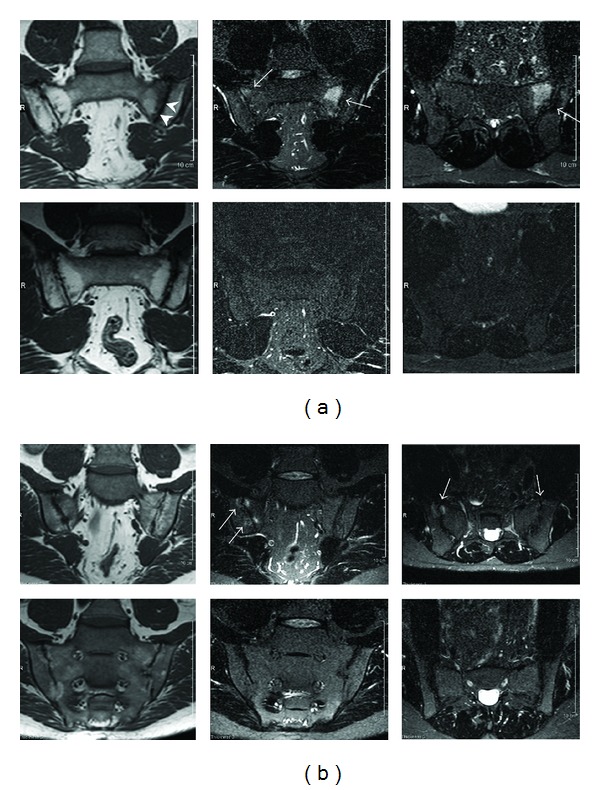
(a) A representative MRI study at inclusion (top row) with bilateral inflammatory changes (arrows). There is a 2 × 3 cm high signal intensity lesion on the sacral side of the left sacroiliac joint on the STIR images (coronal and axial) with corresponding low signal intensity on the coronal T1-weighted image. At the right sacroiliac joint, there are smaller and more subtle lesions on both sides of the joint. There are sclerotic changes along the left joint (arrowheads). At 16 weeks (bottom row), no oedematous lesions can be seen. (b) An MRI study showing bilateral sacroiliitis in an AS patient at inclusion and at 16 weeks. At inclusion, there are bilateral inflammatory changes on the MRI images (top row, arrows). At the right sacroiliac joint, there is a 1 × 1 cm high signal intensity lesion in the sacrum on the STIR images (coronal and axial) with corresponding low signal intensity on the coronal T1-weighted image. On the left, there are smaller and more subtle lesions on both sides of the joint. There are sclerotic changes along the right sacroiliac joint. At 16 weeks (bottom row), no oedematous lesions can be seen.
